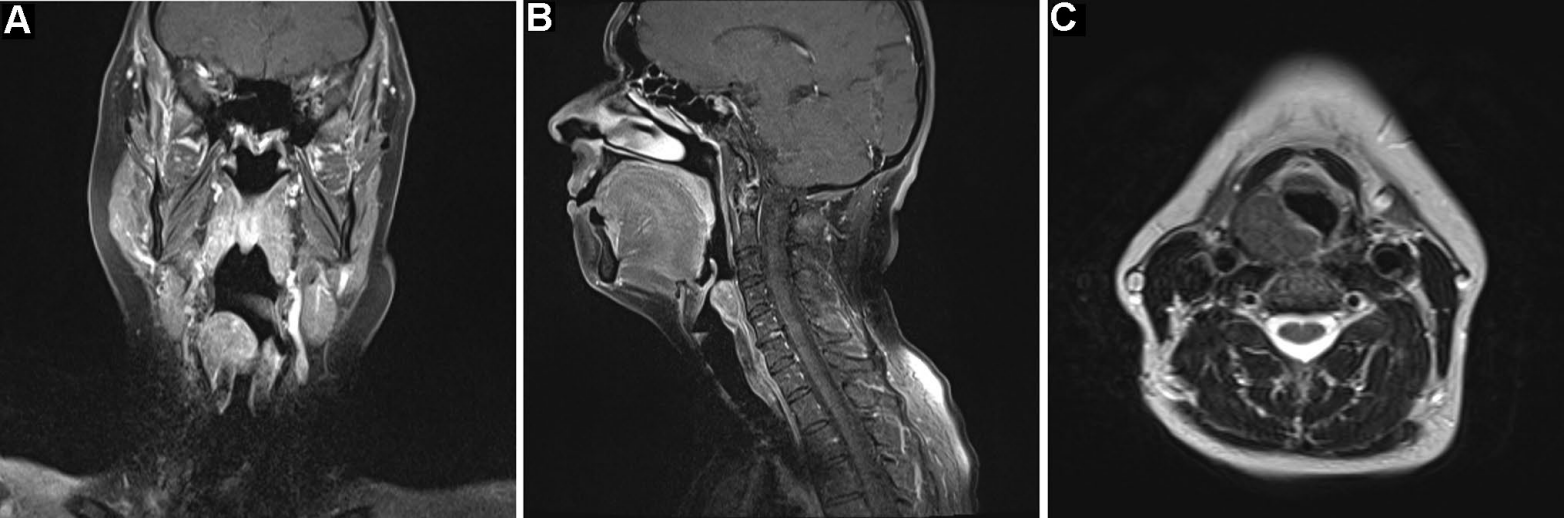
MRI scans with contrast demonstrating an enhancing mass centered on the right piriform fossa a T1-weighted coronal, b T1-weighted sagittal, c T2-weighted axial
